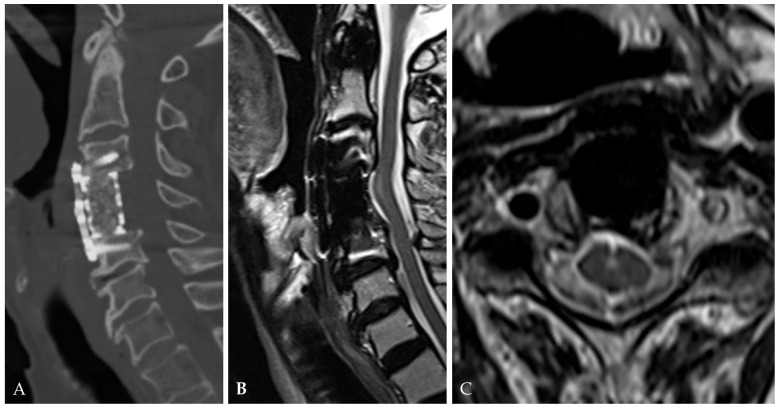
At postoperative 1 month, CT (A) and MRI (B,C) showed complete decompression of the C4–5 OPLL and healing of the dura tear and CSF leakage.
